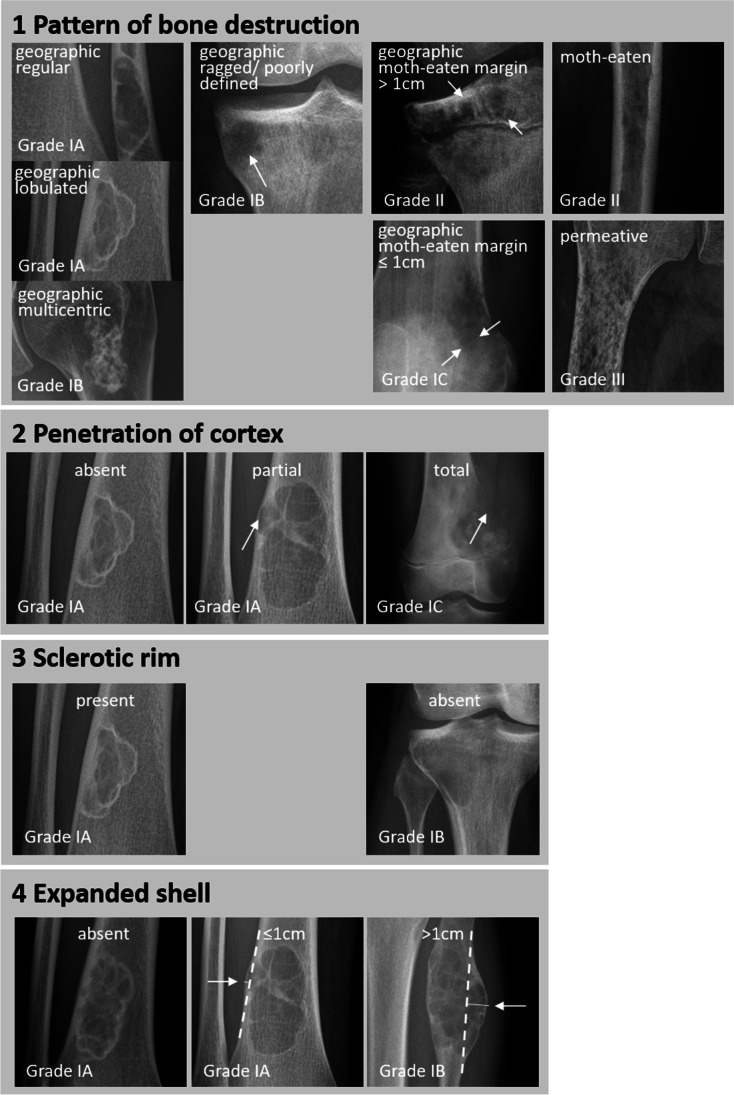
Radiographic examples of the descriptors employed by the Lodwick grading algorithm for bone tumor growth rate
